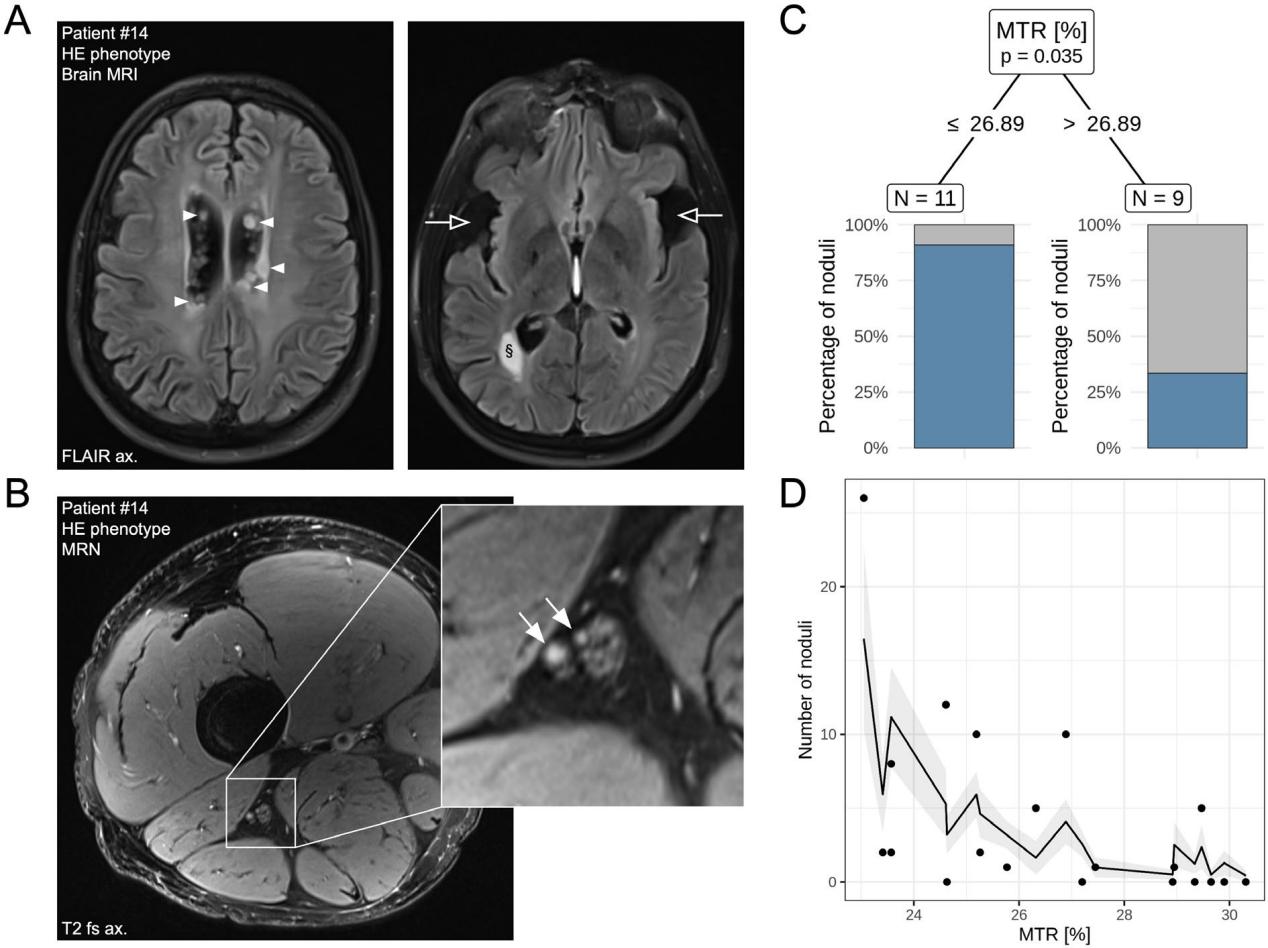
Association between subependymal nodules and peripheral neuropathy in HE patients. (A) Axial FLAIR images of a representative high excretor (HE) patient reveal multiple subependymal nodules along the lateral ventricles (arrowheads), asymmetric focal white matter hyperintensities (§) and fronto‐temporal hypoplasia (open arrow pointing at widened Sylvian fissures). (B) Corresponding axial T2‐weighted MR neurography of the same patient reveals fascicular T2 hyperintensities in the sciatic nerve (solid arrows), consistent with polyneuropathic changes. (C) Binary classification tree based on sciatic nerve MTR values identifies a cut‐off at 26.89%, separating patients with and without subependymal nodules. Nodules were present in 10/11 patients with MTR ≤ 26.89%, compared to 2/9 patients with MTR > 26.89% (*p* = 0.035). (D) Poisson regression analysis demonstrates an inverse relationship between sciatic nerve MTR and the number of subependymal nodules. The black line depicts the fitted model with 95% confidence intervals shown in grey.
